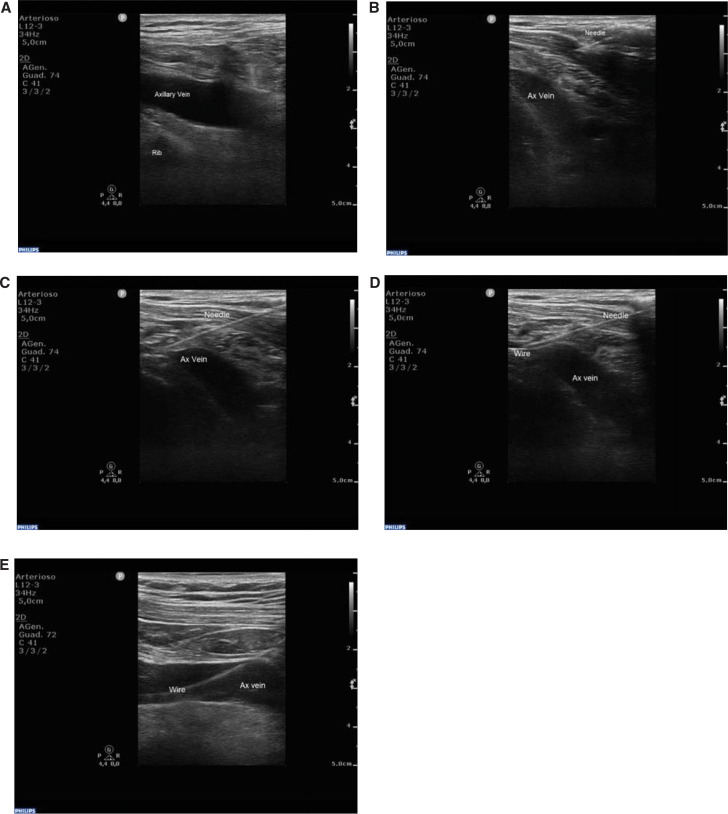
The longitudinal view of the axillary vein allows the operator to track the needle in the subcutis up to the anterior venous wall (A–C), and to monitor insertion of the wire into the vessel (D). Once the needle was seen to enter the vein, the syringe was removed and a 0.035′′ J-tipped guidewire was inserted into the vein and advanced with gentle pressure, without fluoroscopic guidance but under ultrasound guidance (E). *Abbreviation:* Ax, axillary.
